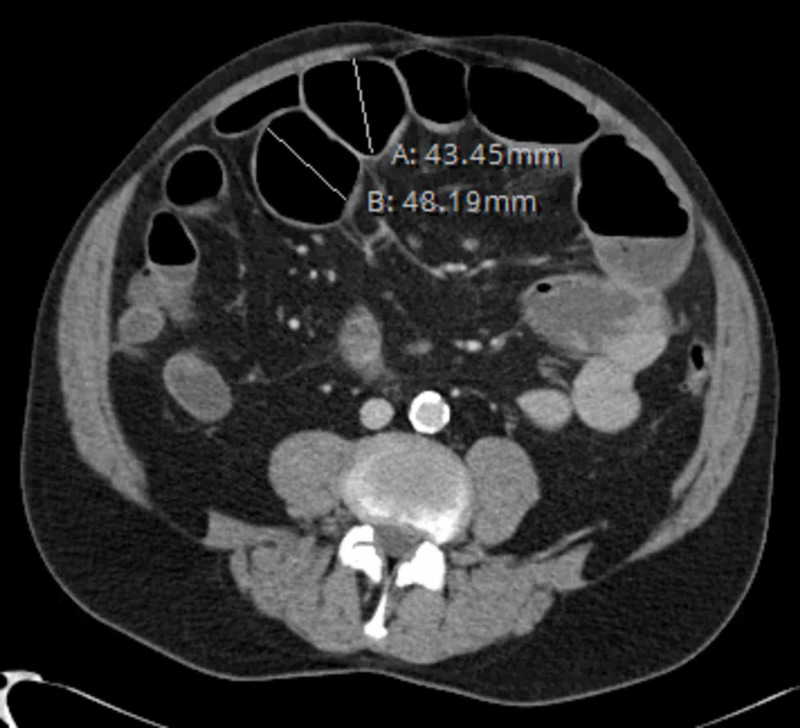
Computed tomography (CT) scan of the abdomen and pelvis showing a high grade small bowel obstruction with the small bowel lumen measuring up to 4.8 cm.
